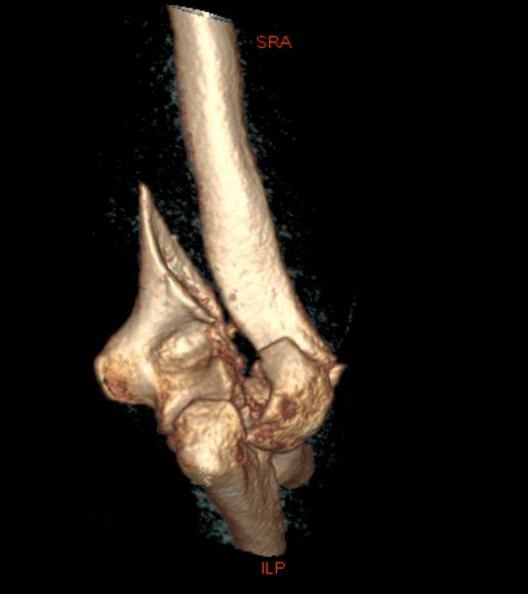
Pre-operative CT and parallel plating of AO type C1 fracture.
